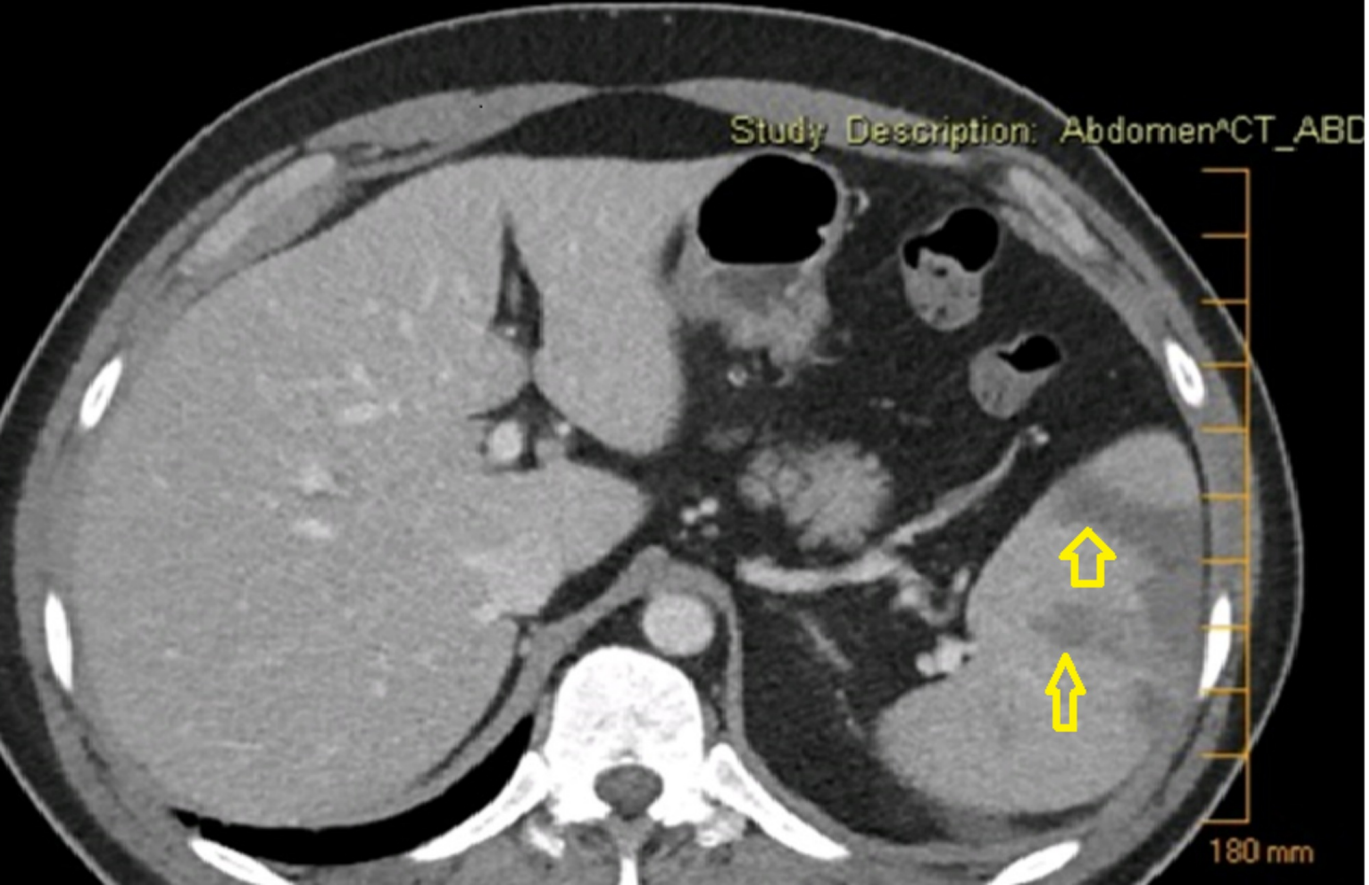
CT abdomen with arrows pointing to splenic infarction CT: computed tomography
